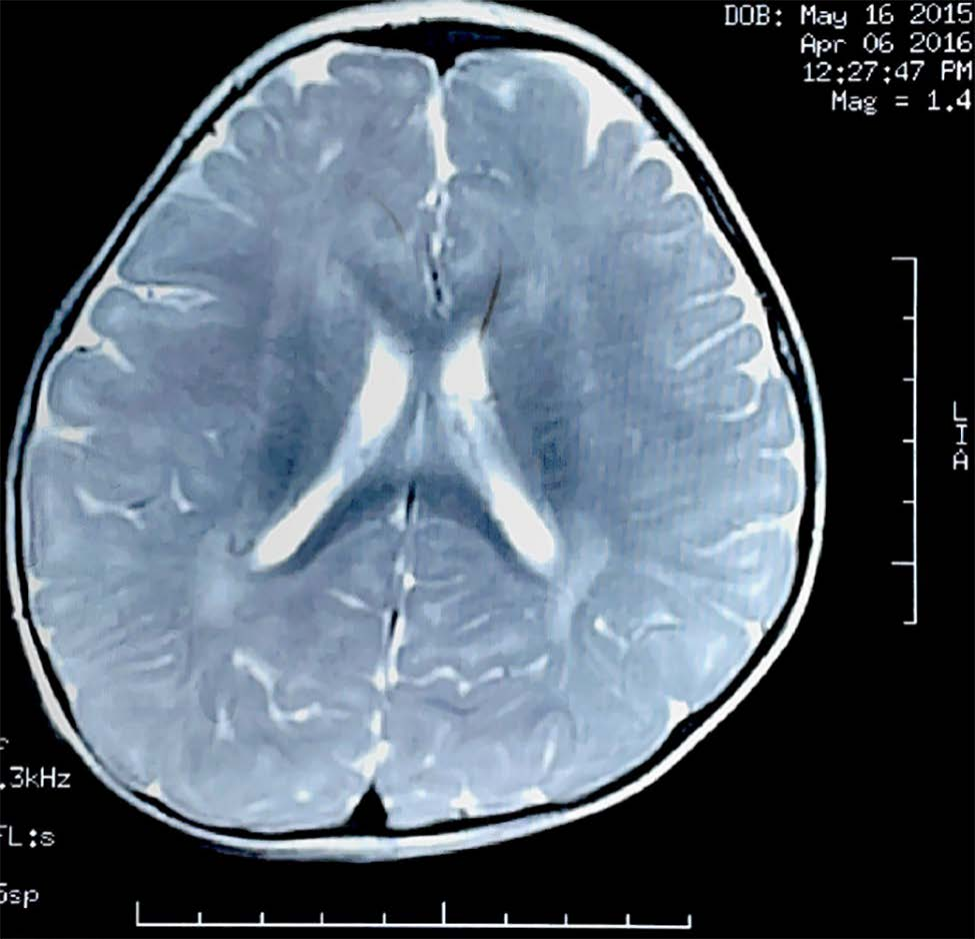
MRI images. Patient at age 11 months: abnormal signal in the white matter around the posterior part of the bilateral lateral ventricles.
